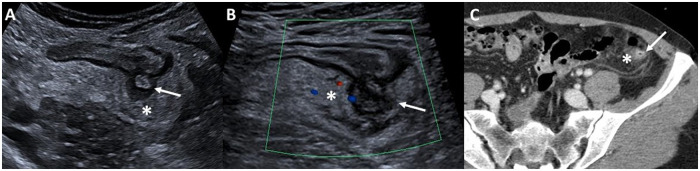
Acute left colonic diverticulitis stage 0. US images obtained with convex transducer (A) and lineal transducer (B) in the axial plane show a mural thickening diverticulum (arrow) with echogenic fat tissue around it (*). A contrast-enhanced CT scan of the same segment (C) shows hyperattenuating mural diverticulum (arrow) surrounded by pericolic inflammation (*).
